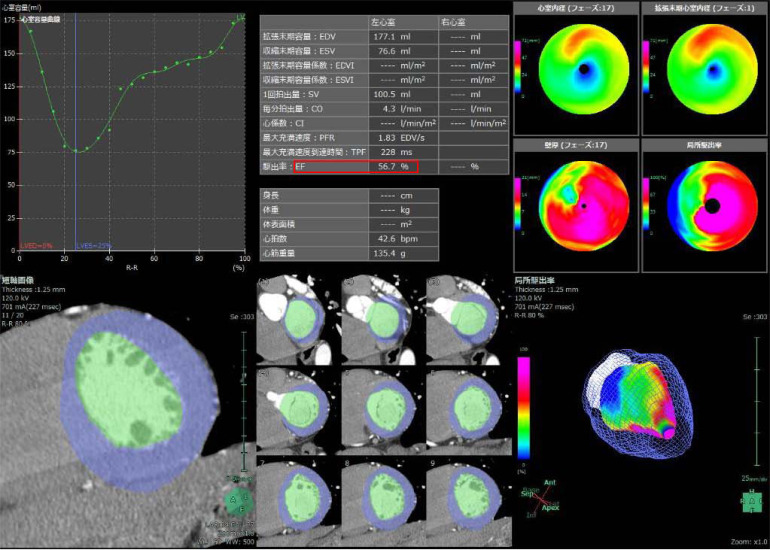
A coronary computed tomography angiography was performed on the same patient referred to in Fig. (1), with pre-study β-blockers (bisoprolol and landiolol) The EF was calculated as 56.7%.”
